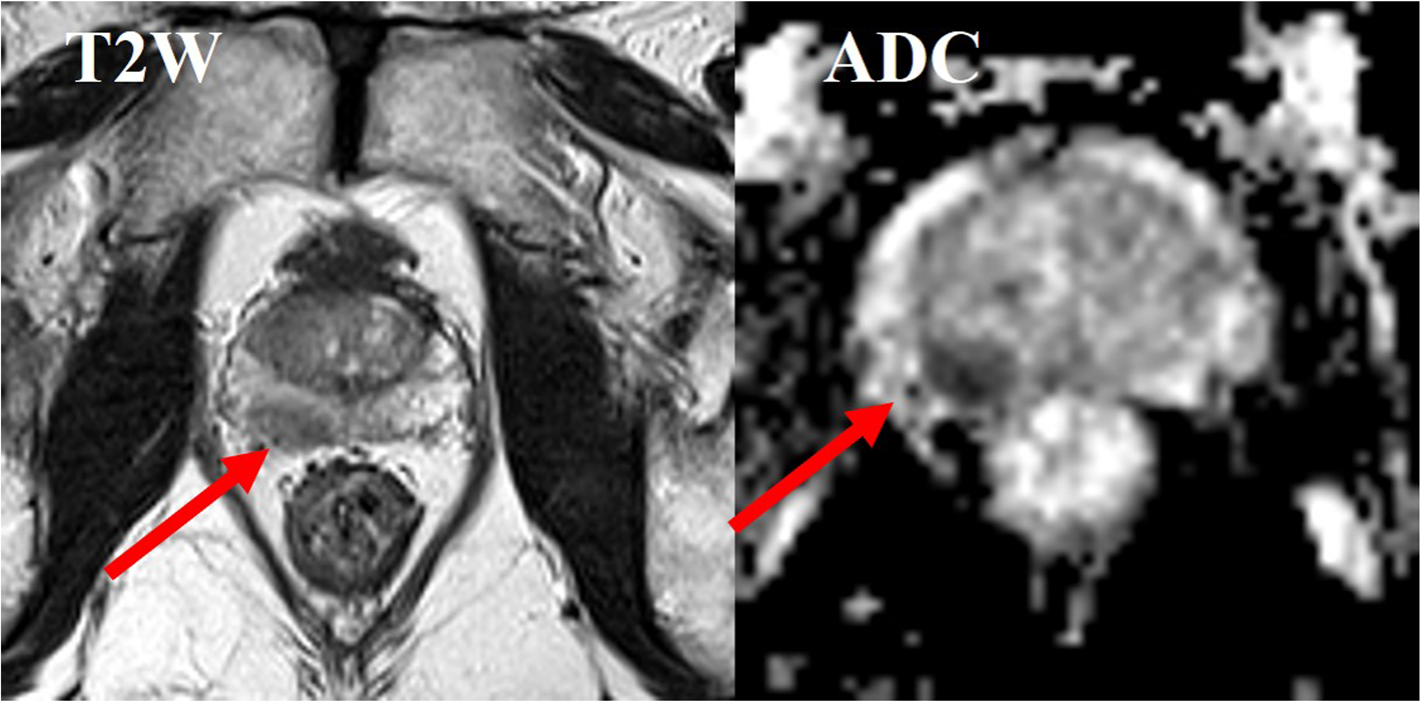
T2-MRI with apparent diffusion coefficient map of DWI
